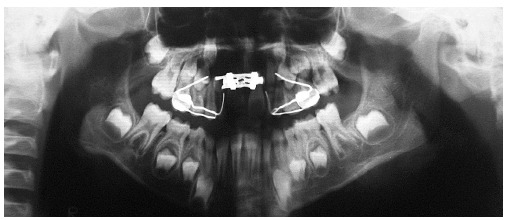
Panoramic radiograph at first treatment phase completion, immediately
